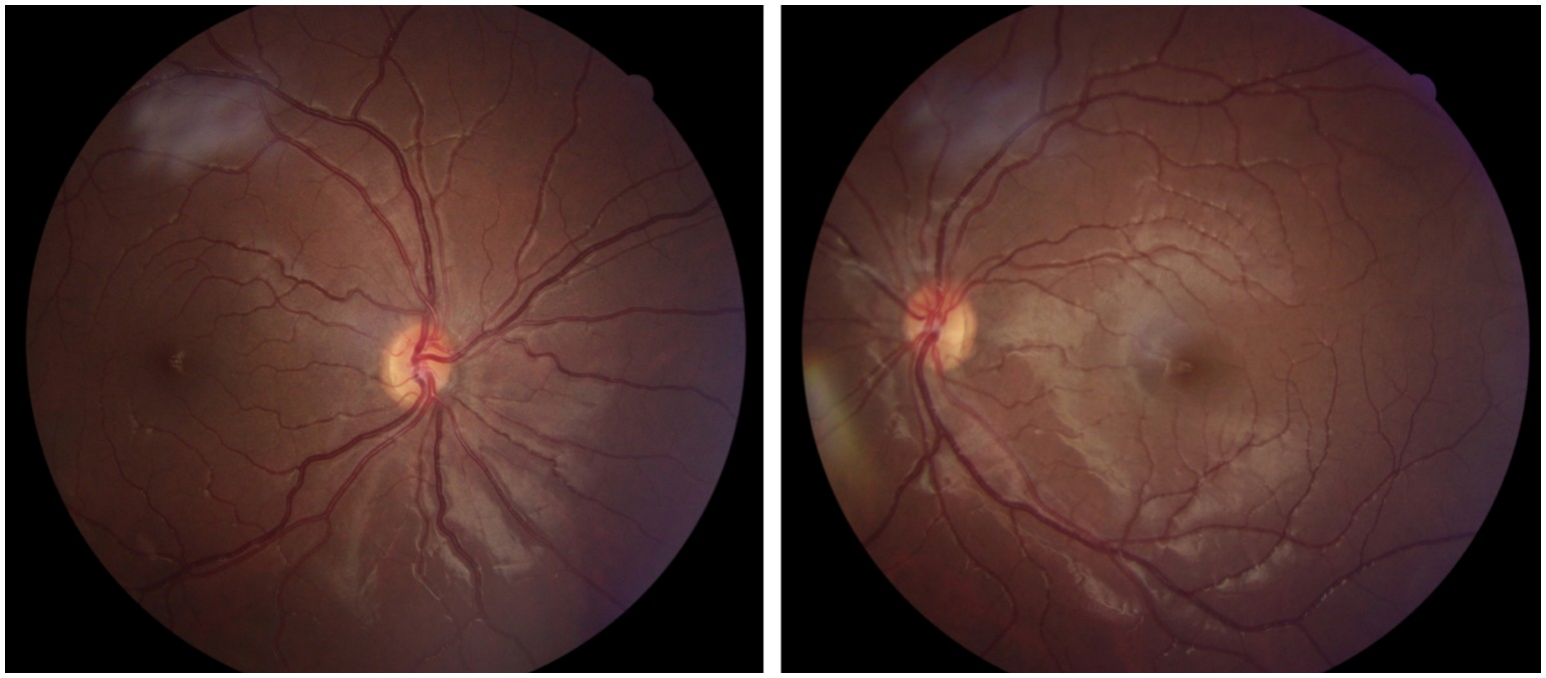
Fundus photographs from Case 29 with bilateral optic nerve hypoplasia. Case 29 was born full term and came to Sweden from Iraq at four years of age. She had strabismus (exophoria left eye) and multiple brain malformations, including thin optic nerves, on magnetic resonance imaging. However, bilateral optic nerve hypoplasia was not diagnosed until she was 17 years of age. At 18 years of age the BCVA was 0.8 right and 0.5 left eye. She was further diagnosed with a moderate intellectual disability and has mild motor impairments.
